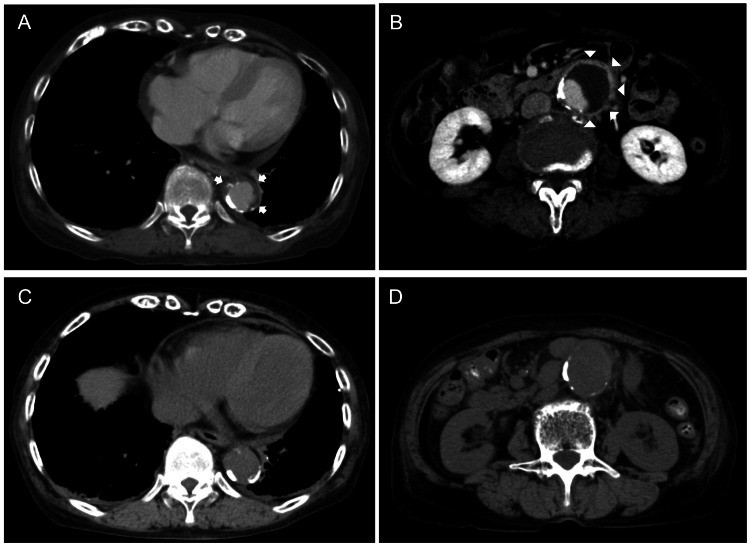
Axial views of the CT angiogram of the thorax and abdomen on admission and plain CT on the third hospital day. A) Descending thoracic aortic arteritis on admission. The arrows show an ill-defined soft tissue abnormality measuring 30 mm × 27 mm. B) Infrarenal abdominal aortic aneurysm on admission. The arrowheads show an ill-defined soft tissue abnormality measuring about 42 mm × 41 mm. C) Descending thoracic aorta on the third hospital day measuring 29 mm × 27 mm. D) Infrarenal abdominal aortic aneurysm on the third hospital day measuring 42 mm × 39 mm. CT, computed tomography.
